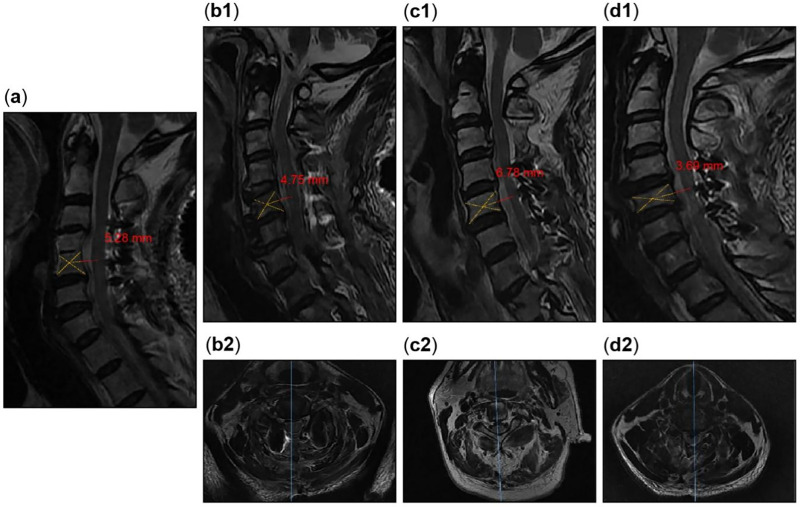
(a) The measurement method for the spinal cord float back distance (SD) on the mid-sagittal MRI. The reference line (yellow dotted line) connected between the center of the vertebral body and its posterior edge. This line was then extended to the center of the spinal cord (red dotted line), and the distance of this extension was defined as SD. The SD was measured at C5 level. (b1,b2) Patients with C5P after total laminectomy: sagittal and coronal MR images. (c1,c2) Patients with C5P after Cervical Posterior Laminoplasty: sagittal and coronal MR images. (d1,d2) Patients without C5P after Cervical Posterior Laminoplasty: sagittal and coronal MR images (This measurement technique is applicable to vertebrae with stable alignment; severe deformities were excluded to maintain reproducibility).
